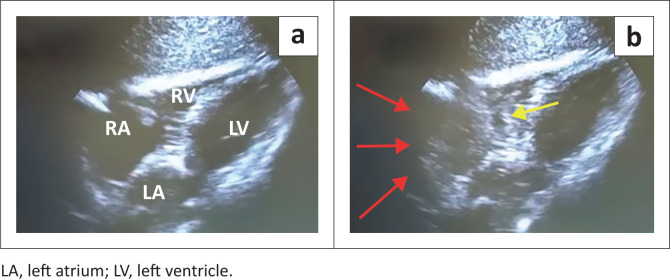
(a) Subcostal ultrasound view of the heart. The empty right atrium (RA) and right ventricle (RV) at the start of the ‘bubble test’. (b) Microbubbles visible within the RA (red arrows) and leading into the RV (yellow arrow) during the ‘bubble test’ at the 1-s mark, indicating correct CVC tip position.
